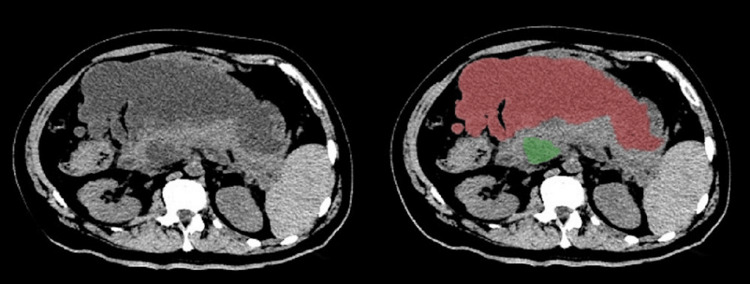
Peripancreatic collections. Abdominal computed tomography showing two peripancreatic collections: anterosuperior (red) and posteroinferior (green).
